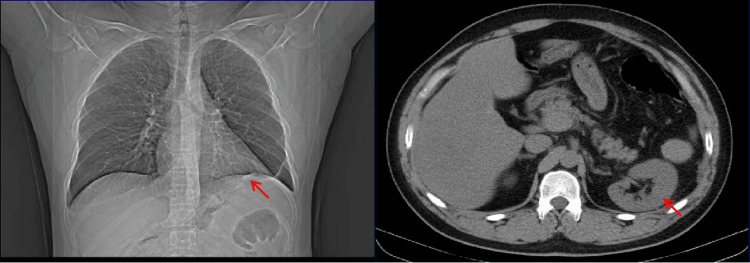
Chest CT at the three-month follow-up. Notable expansion of the left lower lung and the secure fixation of abdominal organs within the abdominal cavity (arrow).
